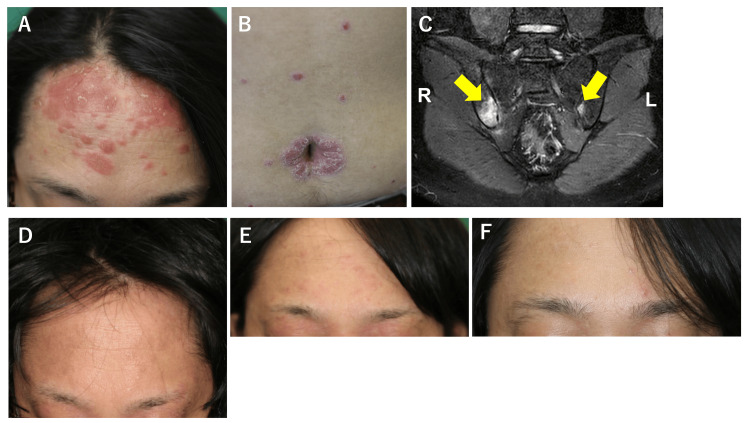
Clinical and radiological findings A, B) Scaly erythematous plaques on the forehead and the abdomen. C) Magnetic resonance imaging with short tau inversion recovery sequences revealing high signal intensity in both sacroiliac joints, with the right side showing more prominent findings (yellow arrows). D) Complete resolution of the skin lesion on the forehead after 12 weeks of treatment with ixekizumab. E) Eczematous lesions on the face after 22 weeks of treatment with ixekizumab. F) Improvement in eczematous lesions 4 weeks after switching to upadacitinib.
